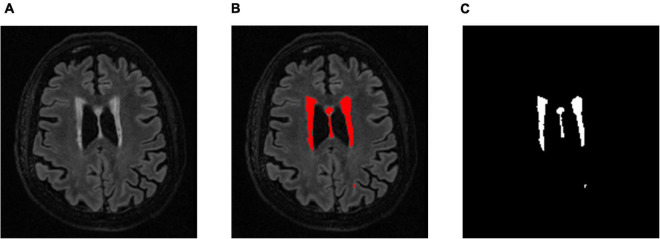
(A) An example a slice of T_2_ FLAIR image volume from one subject showing multiple white matter hyperintensity (WMH) lesions; (B) the results of WMH segmentation using the lesion prediction algorithm (LPA) showing in red, and (C) a WMH binary mask after tissue segmentation, which was used to form the 3D structure of WMH for feature extraction.
